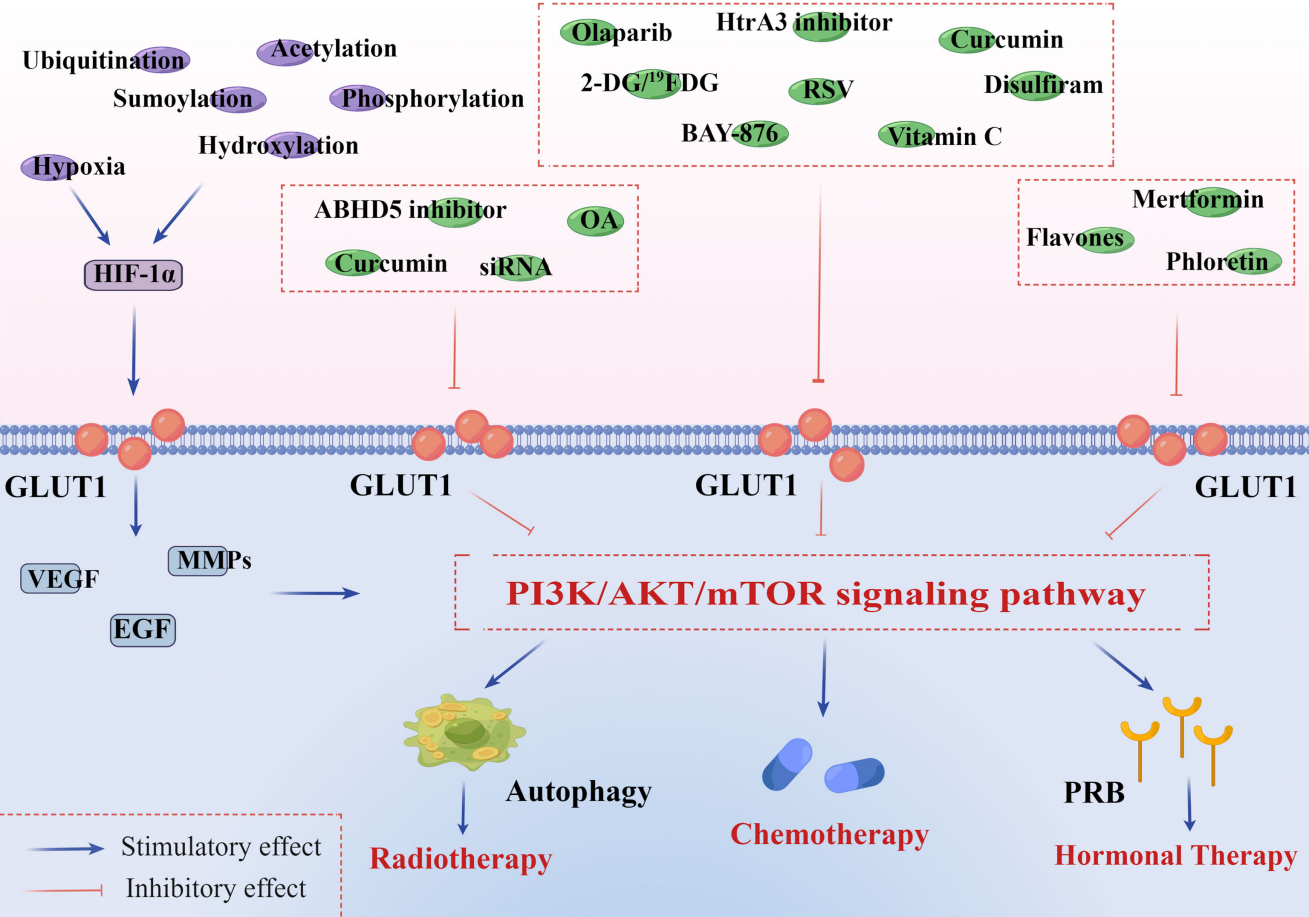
PI3K/AKT/mTOR signaling pathway is associated with GLUT1 overexpression and endometrial cancer therapies. HIF-1α is implicated in both GLUT1 expression and aberrant PI3K/AKT/mTOR signaling pathway in tumor microenvironments. In addition to hypoxia, HIF-1α can be constitutively induced by oxygen-insensitive pathways, such as ubiquitination, acetylation, sumoylation, hydroxylation, and phosphorylation. These pathways jointly promote the expression of HIF-1α and further activate the downstream genes of GLUT1, including VEGF, EGF, and MMPs. 1) Radiotherapy: curcumin inhibits a site overlapping the cytochalasin B of GLUT1 and metabolism-related enzymes. OA decreases the expression of many significant factors, including GLUT1, HIF-1α, Ki67, and P53. GLUT1-siRNA interferes with the targeted gene, inhibiting the synthesis of GLUT1. ABHD 5 plays an oncogenic role in the development of EC, and its knockdown can notably suppress ECC proliferation and invasion *in vivo*. All of them can inhibit the PI3K/AKT/mTOR pathway and activate autophagy of endometrial cancer cells (ECCs), increasing the sensitivity to radiotherapy. 2) Chemotherapy: olaparib inhibits the activity of GLUT1 in plasma in a concentration-dependent manner. BAY-876 can suppress cell viability and decrease stemness oncogene (Nanog and c-Myc) expression of ECCs. RSV inhibits GLUT1-induced glycolysis in a PI3K/AKT/mTOR-dependent manner, enhancing the anti-endometrial cancer (anti-EC) effects of cisplatin and doxorubicin. Curcumin inhibits a site overlapping the cytochalasin B of GLUT1 and metabolism-related enzymes. Vitamin C inhibits the expression of HIF-1α and GLUT1 in ECCs. 2-DG/^19^FDG and disulfiram show notably antiproliferative and anti-angiogenesis effects by downregulating the level of GLUT1. Targeting HtrA3 can enhance the cytotoxic effect of chemotherapy *via* the X-linked inhibitor of apoptosis protein cleavage. 3) Hormonal therapy: GLUT1 participates in regulating PR of ECCs in a PI3K/AKT/mTOR pathway-dependent manner. Flavones, phloretin, and metformin can greatly increase the sensitivity of hormonal therapy in EC by strengthening PR transcriptional activity. This figure was drawn by Figdraw (www.figdraw.com). GLUT1, glucose transporter 1; HIF-1α, hypoxia-inducible factor-1α; VEGF, vascular and epidermal growth factor; EGF, epidermal growth factor; MMPs, matrix metalloproteinases; OA, oleanolic acid; siRNA, small interfering RNA; ABHD 5, abhydrolase domain containing 5; RSV, resveratrol; DG, deoxyglucose; PR, progesterone receptor.
